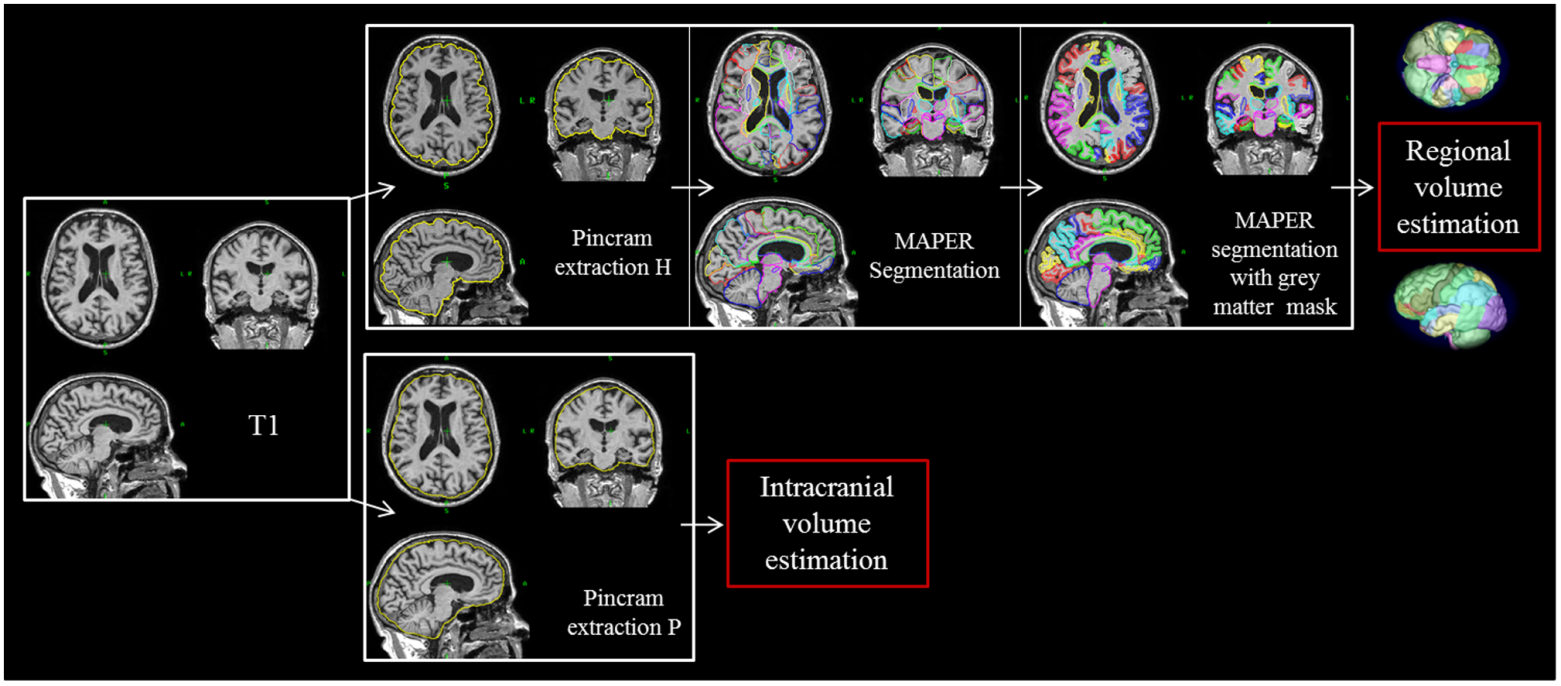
Pincram brain extraction and MAPER segmentation. MRI T1 sequences were processed with pincram, followed by MAPER and grey matter masking to obtain regional grey matter volumes. Extraction P was used to estimate the intracranial volume. *Pincram extraction*
